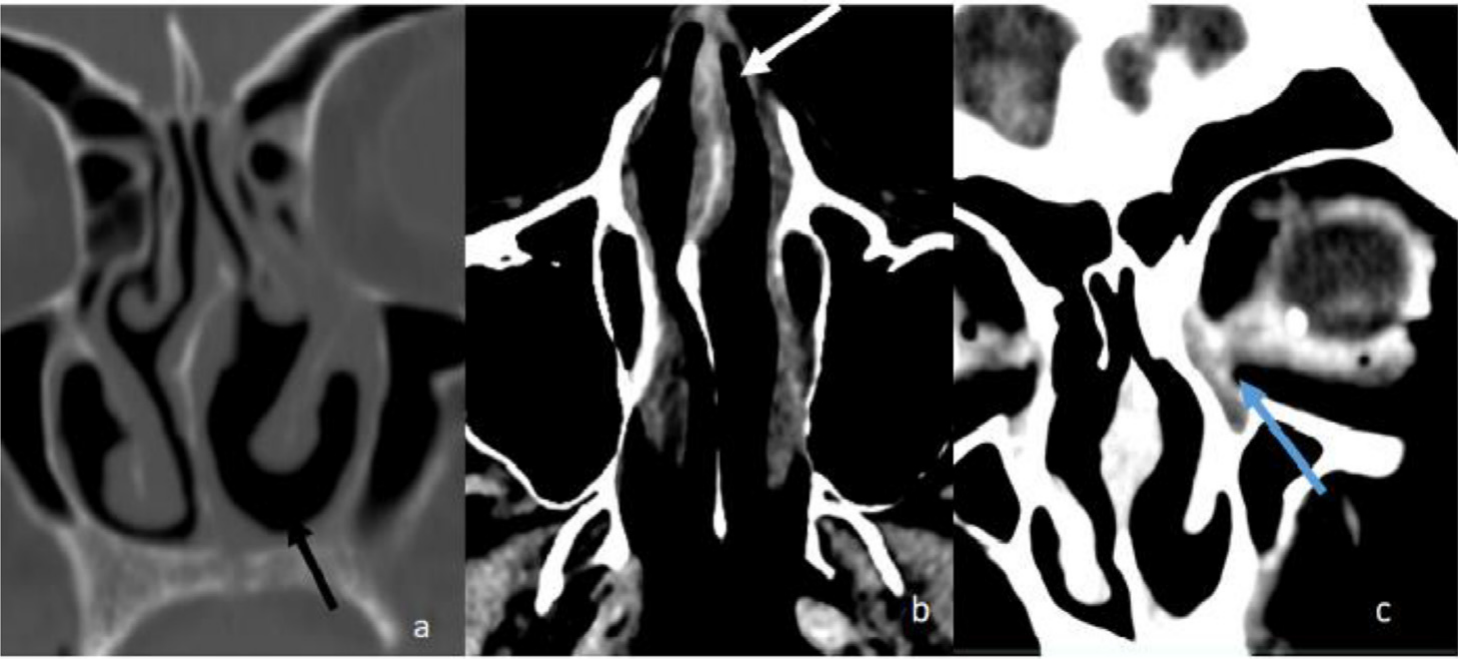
Follow up CT scan 3 months after treatment. (A) Coronal bone window at the level of the inferior turbinate demonstrates complete resolution of the left nasal cavity mass (B) Axial soft tissue window at the same level with patent left nasal cavity and (C) Coronal soft tissue demonstrates complete resolution of the left orbital component.
